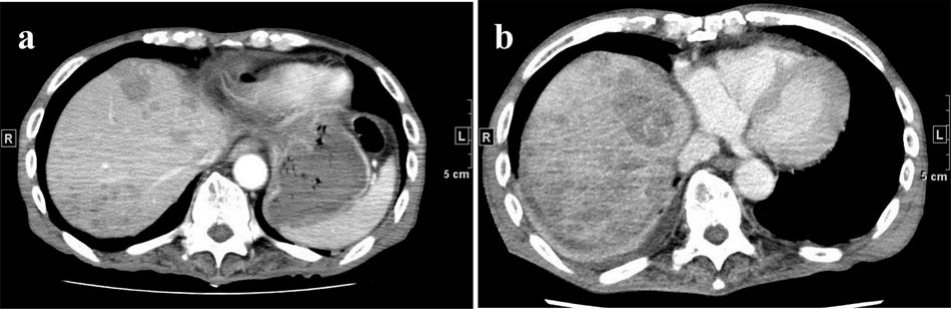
(a) CT on Day 21 after surgery. Multiple tumors are found in the liver. (b) CT re-examination performed 27 days after the operation shows multiple rapidly increasing liver masses. CT, computed tomography.
